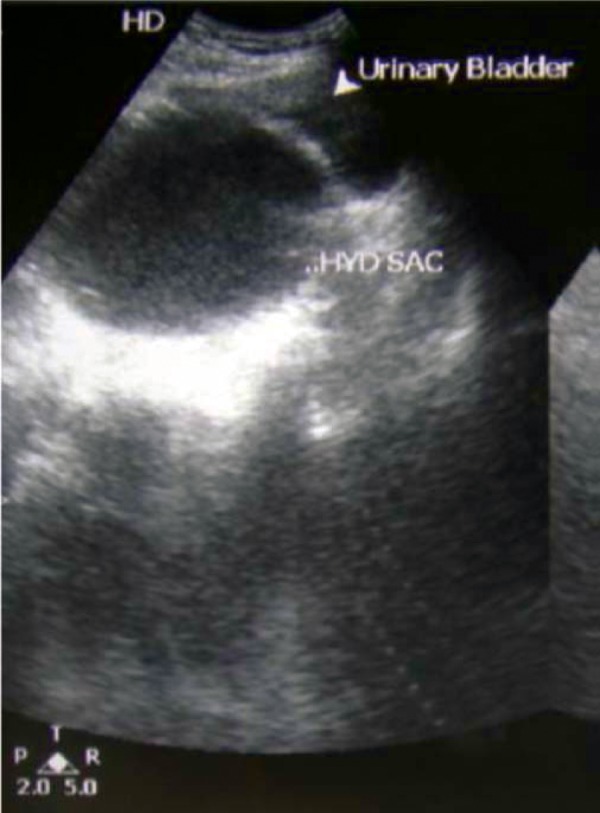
Cystic Lesion Posterior to the Bladder on Ultrasonography
